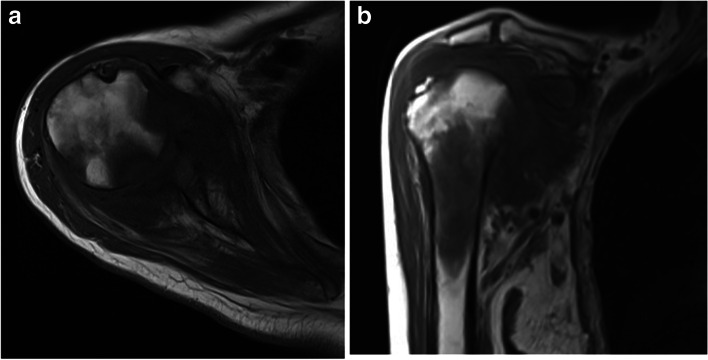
Axial (A) and coronal (B) MR imaging of a right shoulder in a patient with delayed diagnosis and treatment of shoulder sepsis. Findings reveal osteomyelitis of both the humeral head and glenoid vault and secondary arthritis
